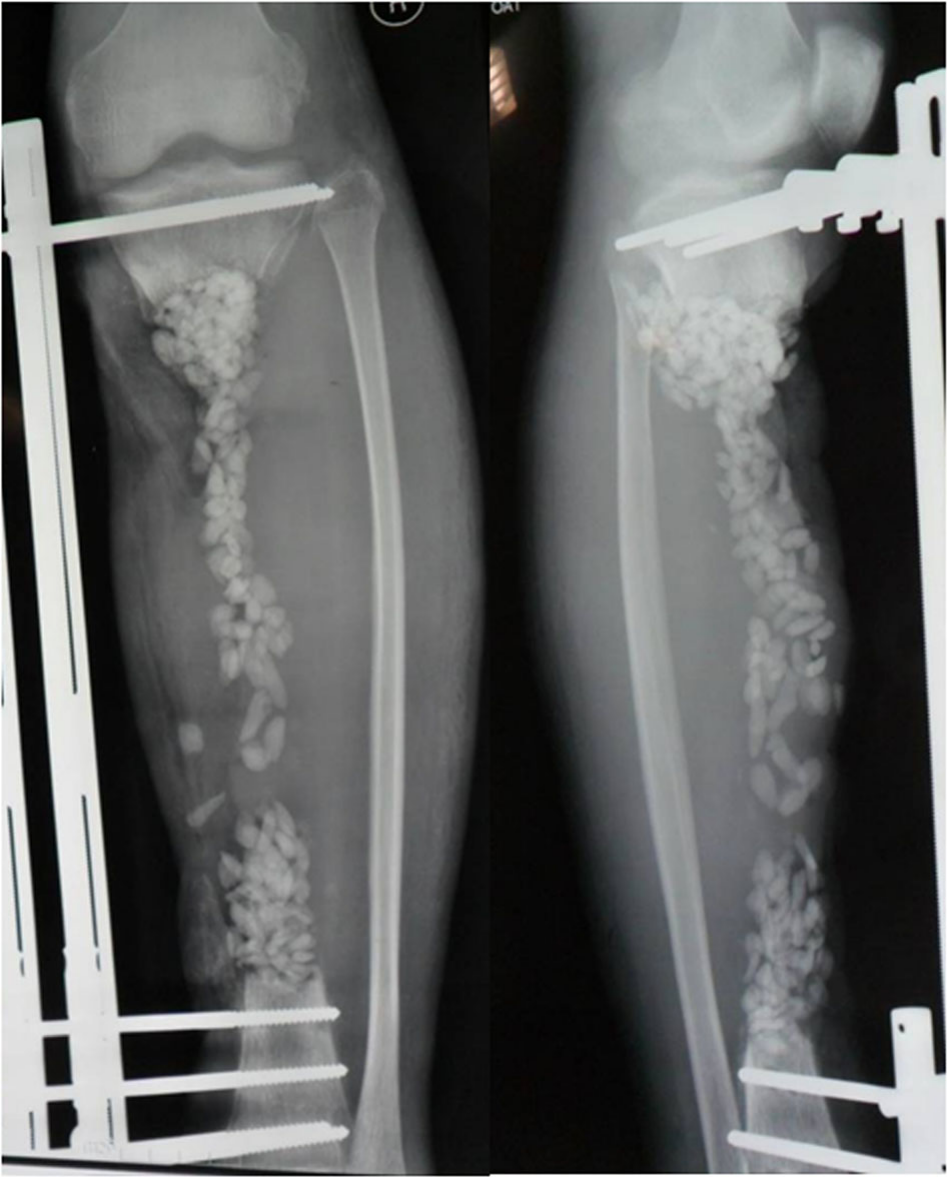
X-ray showing the vancomycin-impregnated cement beads that were placed during the first debridement
